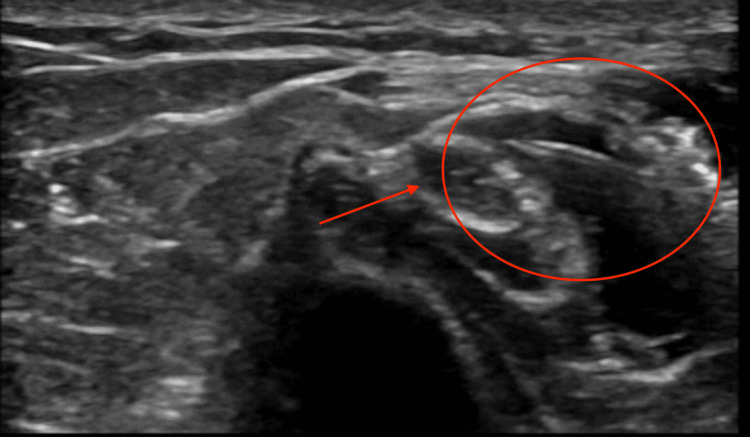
Ultrasound-guided hydrodissection of the ulnar nerve at the cubital tunnel Ultrasound imaging depicts the injection of dextrose and dexamethasone with subsequent hydrodissection of the ulnar nerve away from surrounding tissues.
